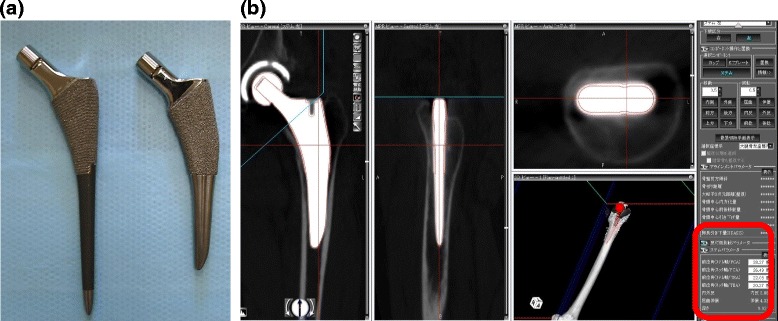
Materials. (a) Photographs of two different types of stem. Left: Summit stem (straight stem), right: TriLock stem (tapered wedge stem). (b) Postoperative CT data were transferred to the planning module and reconstructed in the axial, frontal, and sagittal planes. The computer-aided design model of the femoral implant was superimposed. The parameters of stem alignment are indicated by red circles.
